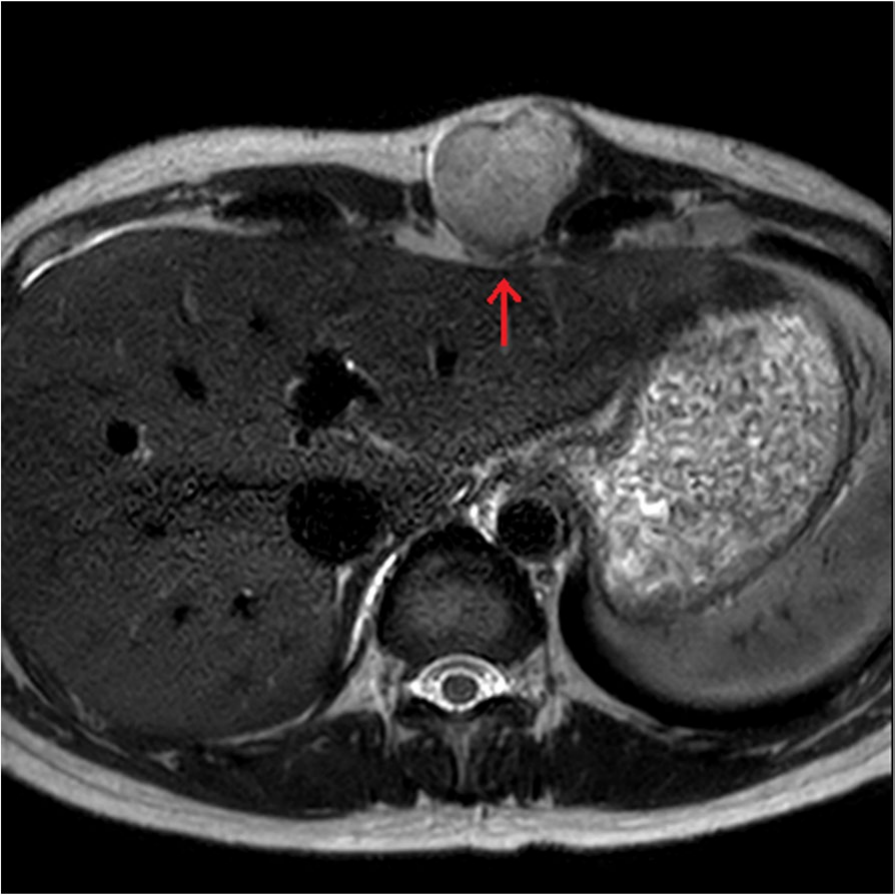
MRI showed a lobulated mass (4.1 × 3.8 × 4.8 cm) in the left paramedian anterior chest wall. MRI revealed the invasion of rectus abdominis muscle (red arrow)
